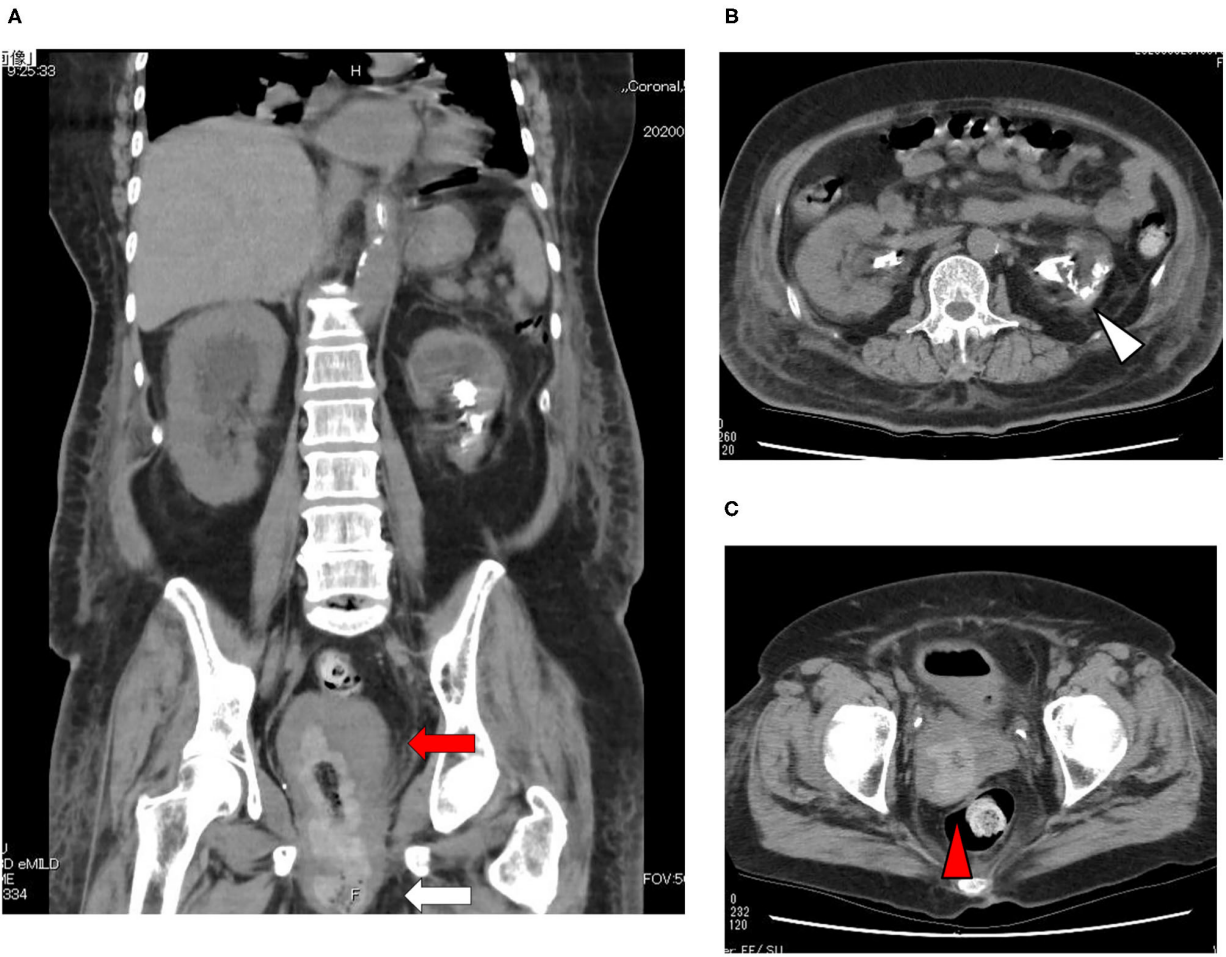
(A–C) Abdominal and pelvic CT taken 10 days after admission. Abdominal and pelvic CT revealed that hydronephrosis was improved, although renal calculus was observed. In addition, pelvic CT revealed that lower shift of bladder (red arrow) and severe uterine prolapse (white arrow) were improved.
